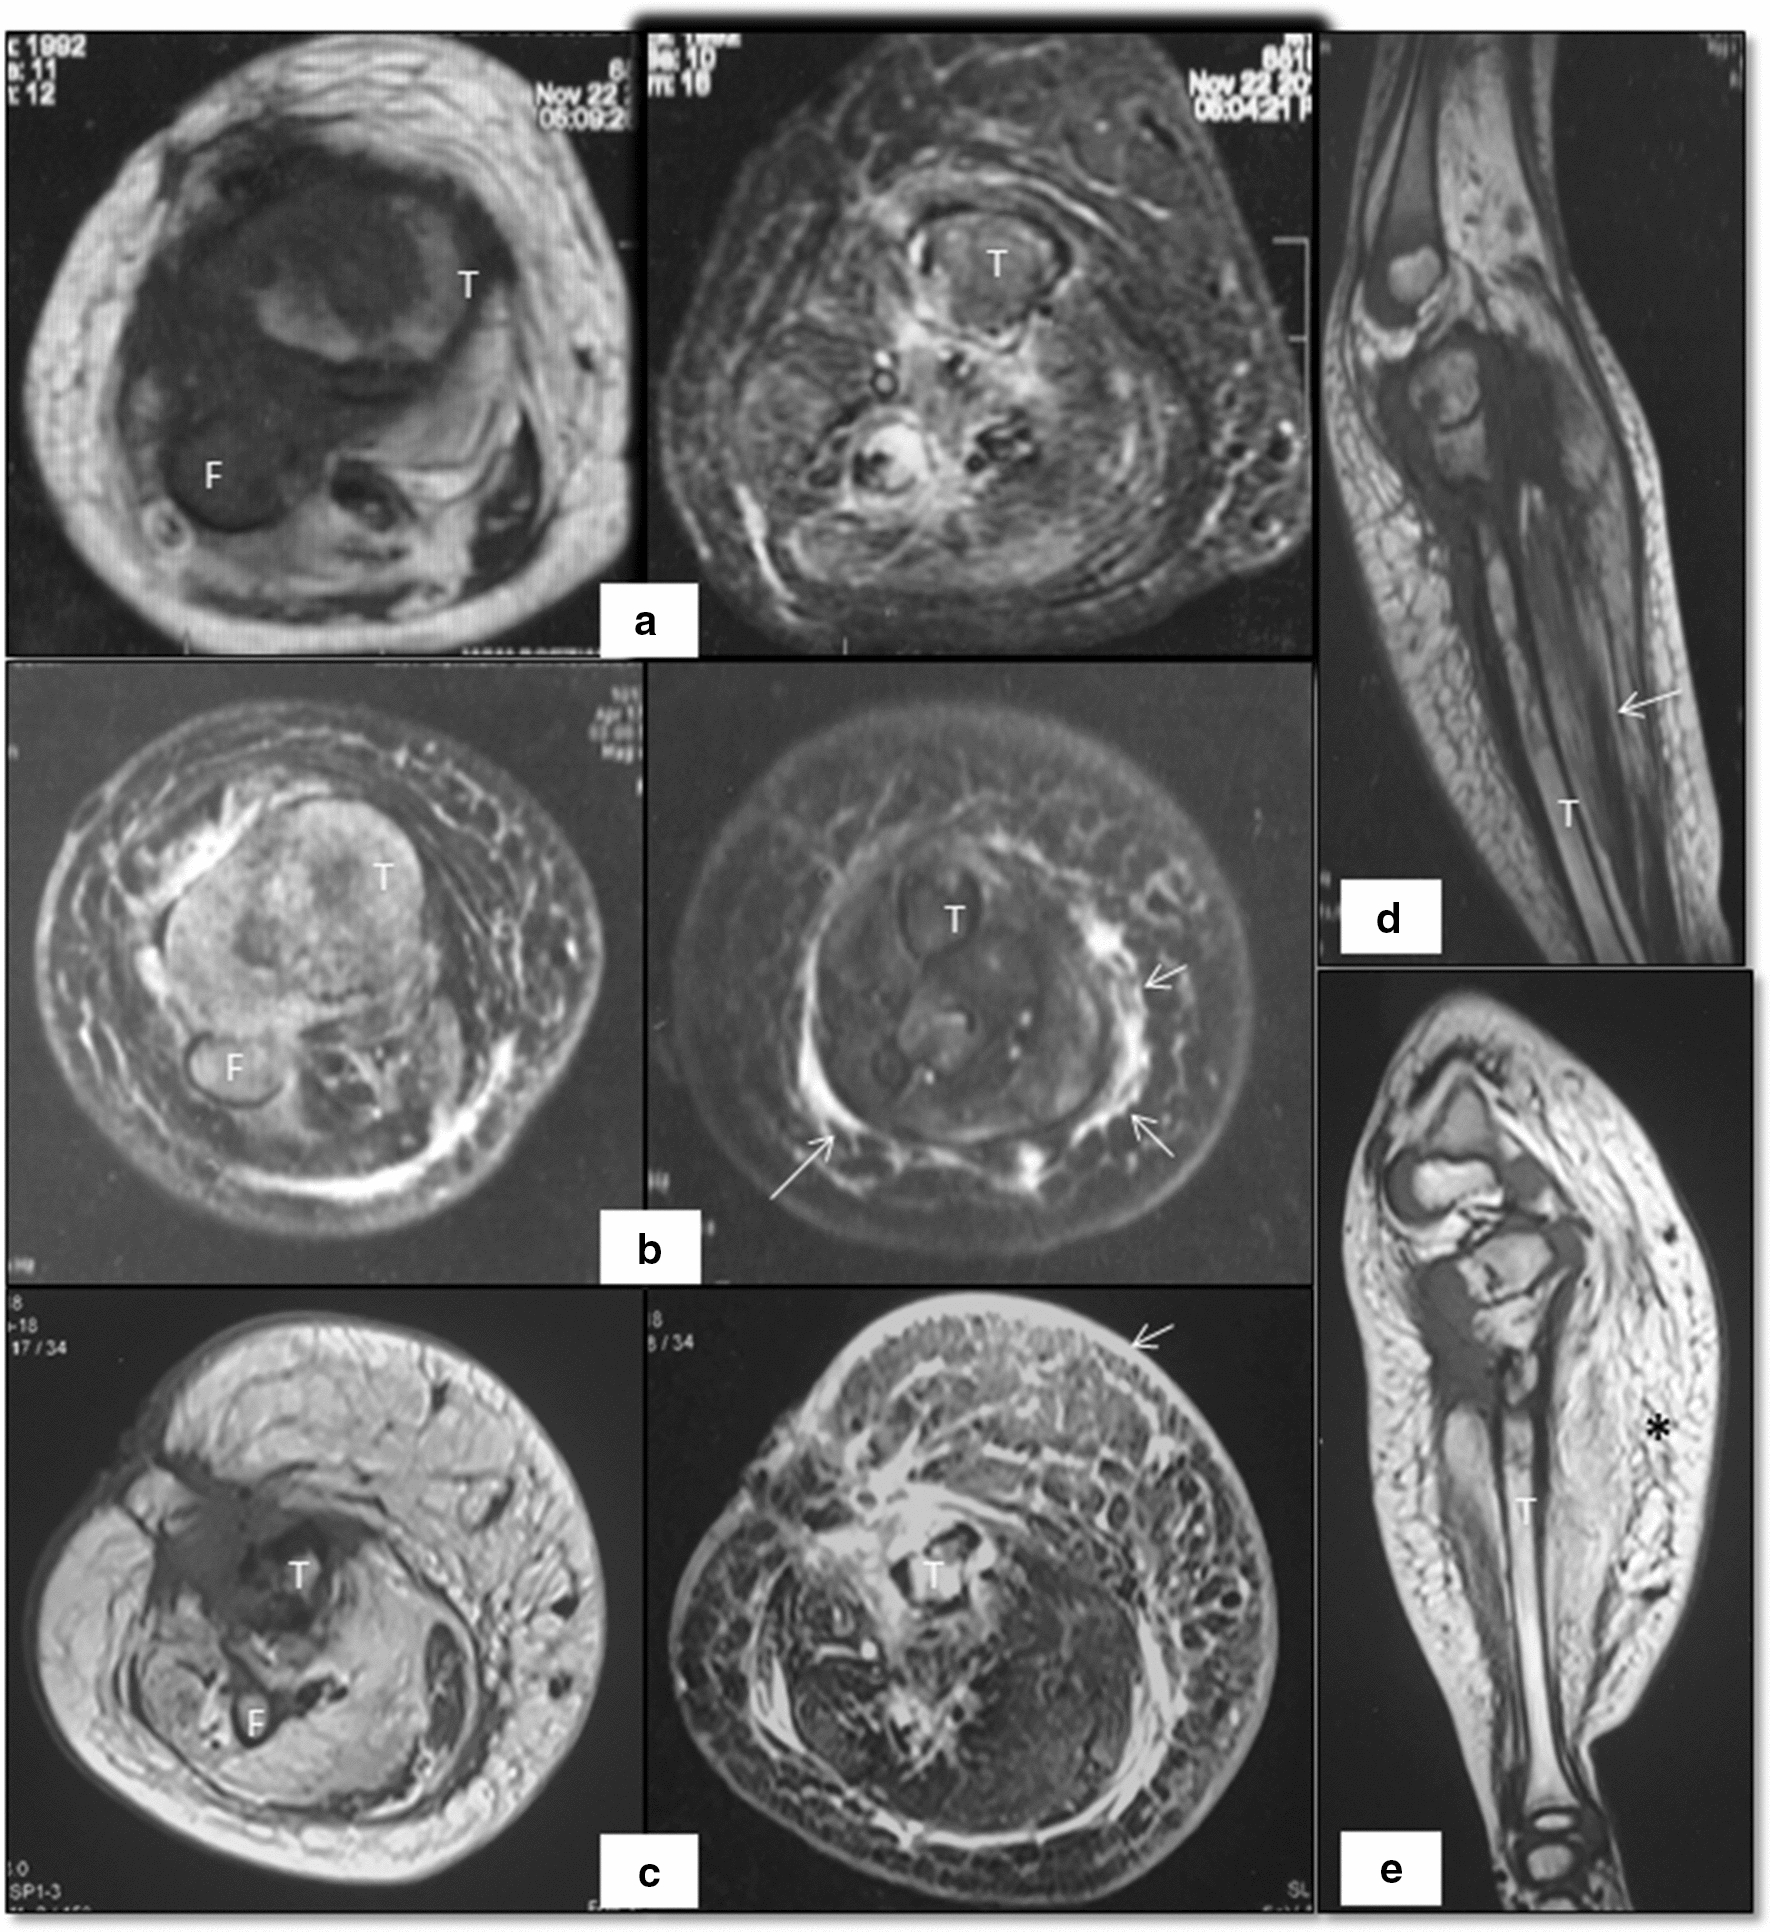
Sequential MRI of patient with Kaposiform haemangioendothelioma. Initial axial MR images a of 2016 show cortical destruction, marrow oedema involving tibia (T) and fibula (F) with surrounding soft tissue oedema on T1 (left) as well as STIR (right) sequences. Subsequently performed MRI in 2017 reveals increase in calf diameter and marrow oedema in both bones with visualization of plaque like hyperintensity along the muscle plane on STIR images (arrows in b). The recent MR images demonstrate trans-compartment involvement of skin (arrow in c) as thickening and hyperintense signal intensity; subcutaneous plane as fat proliferation which shows reticular pattern/lymphedema; muscles as atrophy and fat proliferation within the intermuscular plane and bones with marrow signal alteration. The similar changes are also evident on T1 weighted sagittal images of 2016 (arrow in d) and 2018 (asterisk in e)
